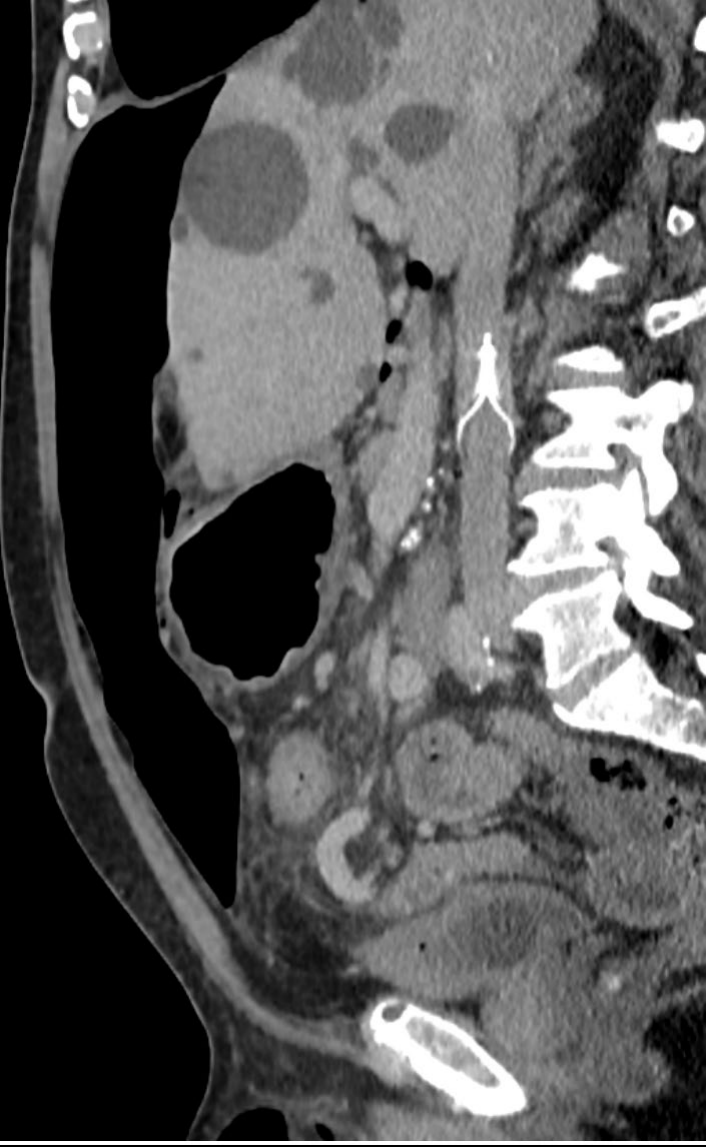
CT reformatted in the sagittal plane showing pneumoperitoneum and IVC filter
